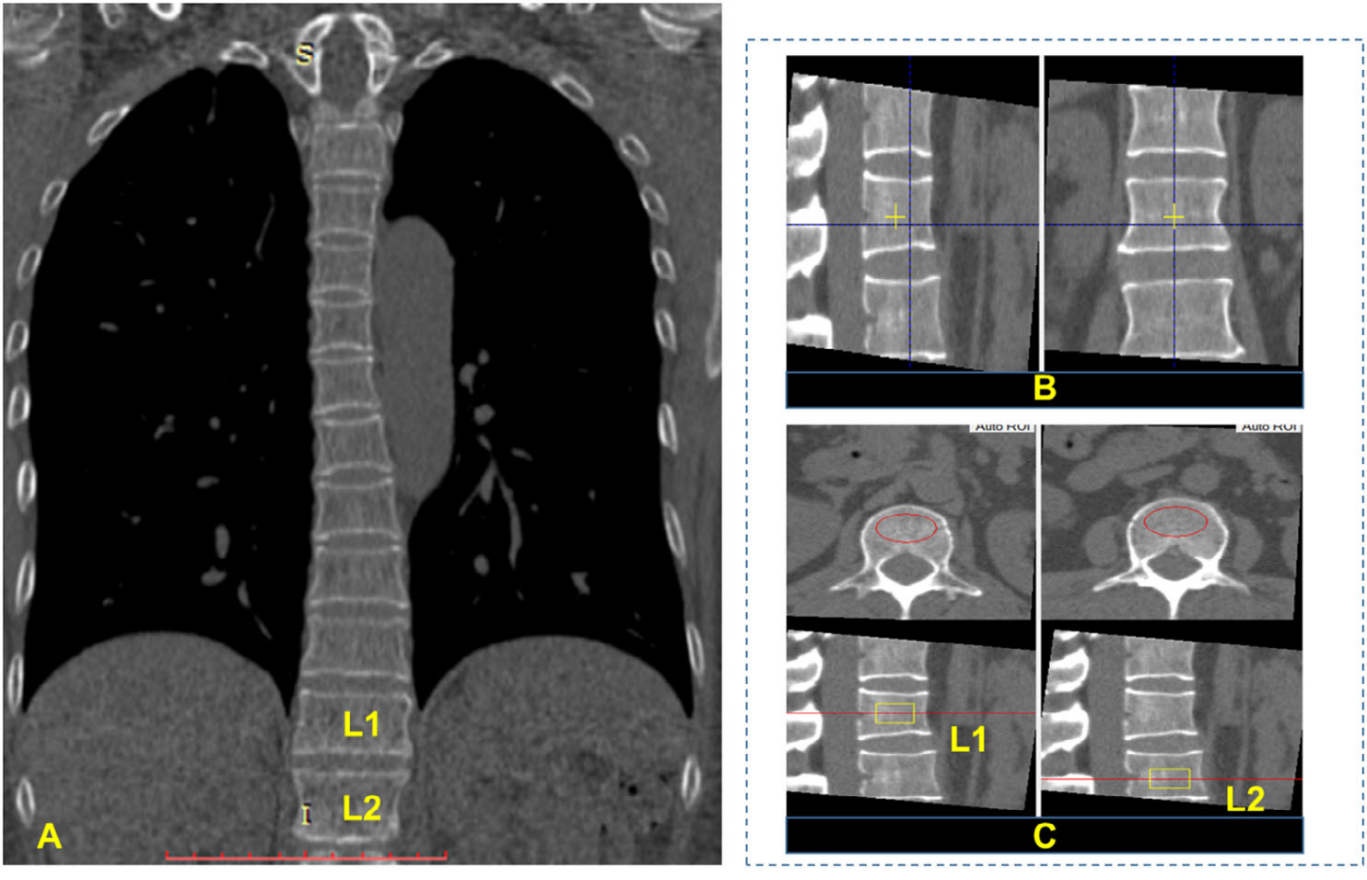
Measurement of vBMD of L1 and L2 with Mindways QCT Pro system. (*A*) Coronal view of a chest LDCT scan. (*B*) Positioning of sagittal and axial views for subsequent automatic placement of analysis VOIs. (*C*) Analysis VOIs shown as red ellipse in axial view and yellow rectangle in sagittal view. LDCT = low‐dose CT; VOI = volume of interest.
